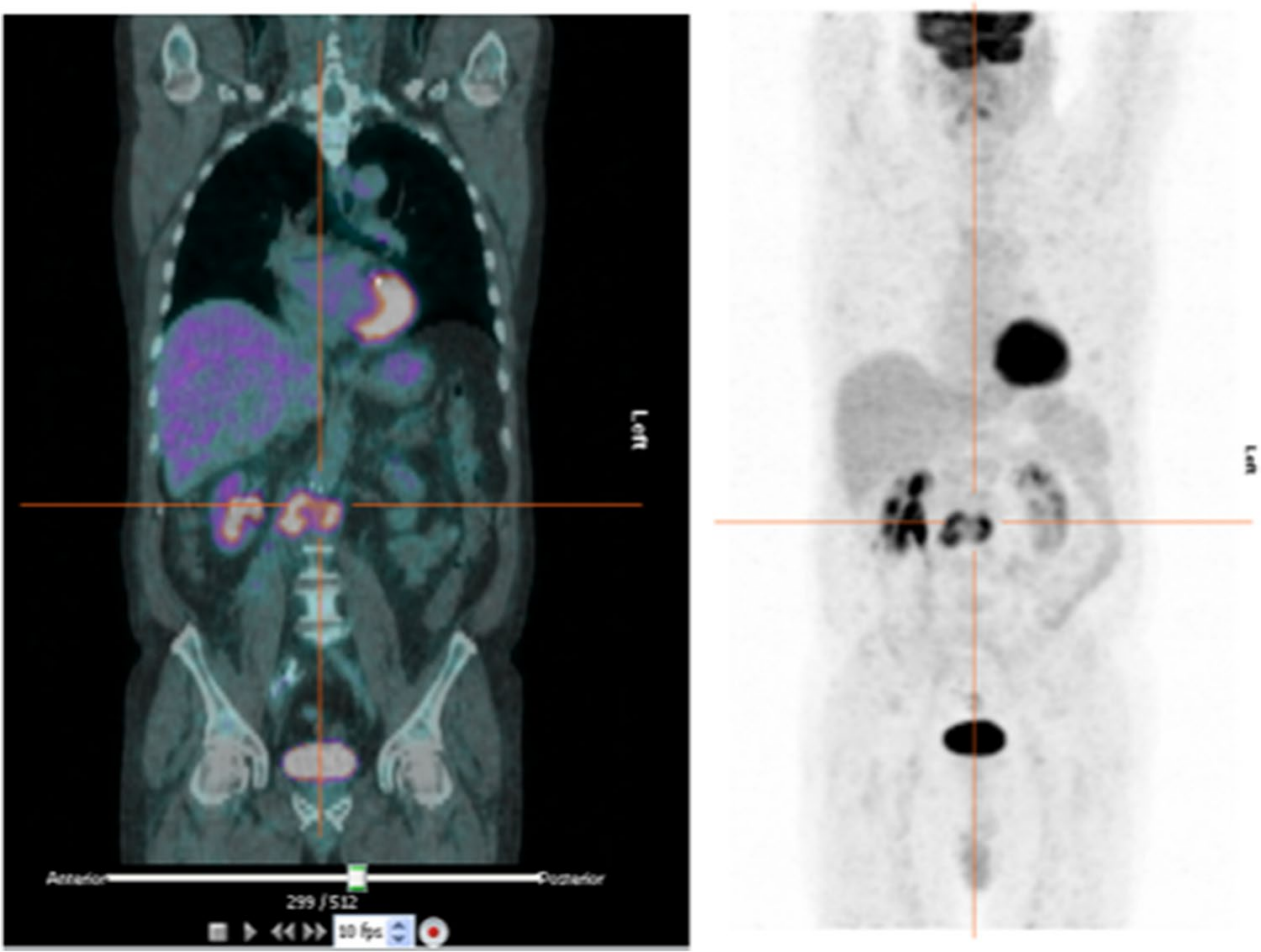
Coronal images of [^18^F]FDG PET/CT (left panel) and MIP (right panel) show focal and intense [^18^F]FDG uptake on the abdominal graft, that is consistent with an infection
